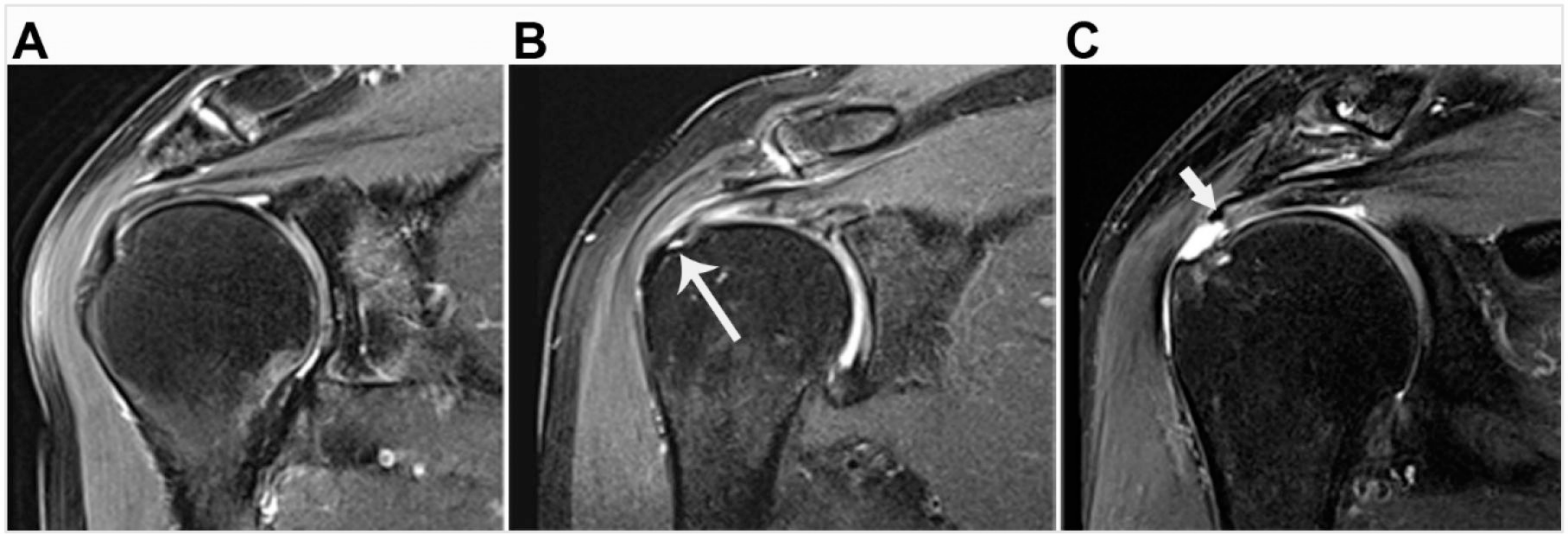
Examples of rotator cuff tendon classification on magnetic resonance imaging. (A) Supraspinatus tendon shows no sign of tear. (B) Partial-thickness tear of the supraspinatus tendon at its greater tuberosity humeral footprint (long arrow). (C) Full-thickness tear of the supraspinatus tendon with medial retraction of the tendon edge (short arrow) away from the greater tuberosity humeral footprint.
